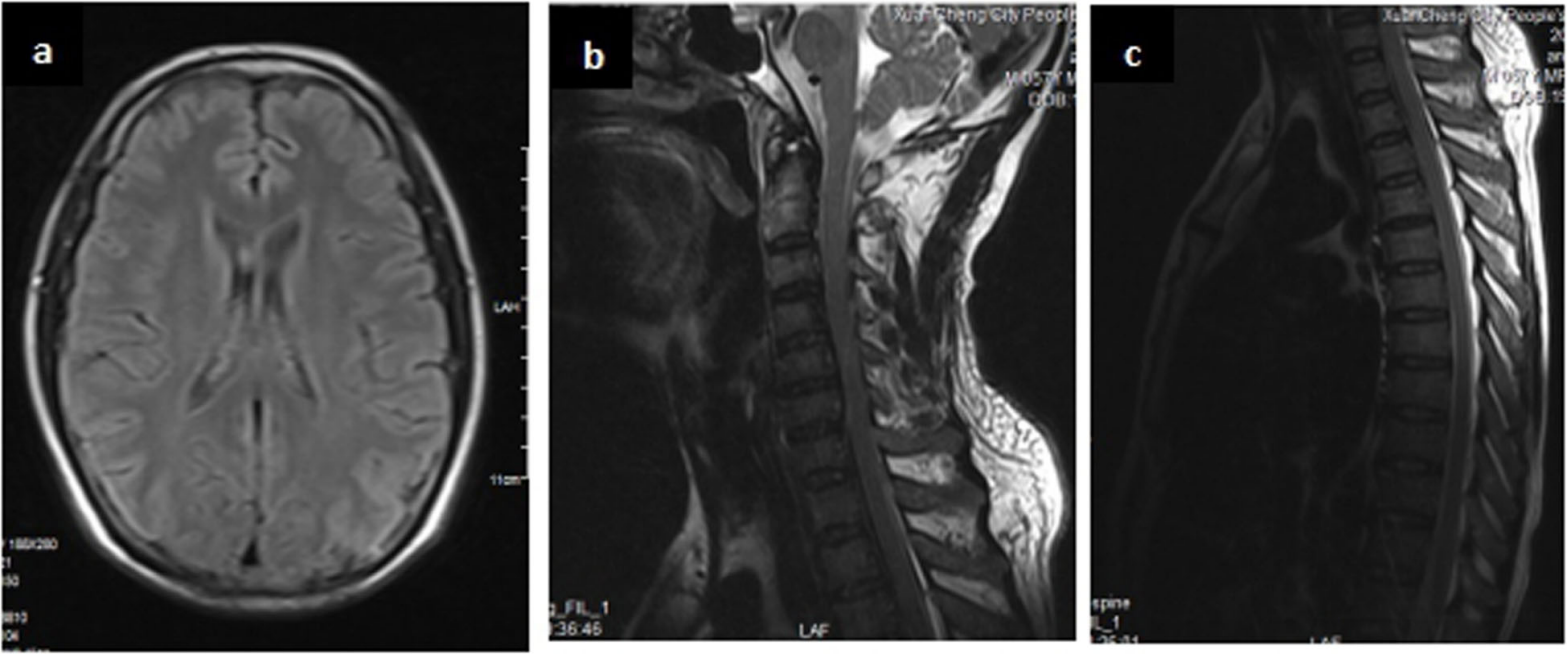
T2-weighted MRI showing normal Brain (a), cervical (b) and thoracic (c)
